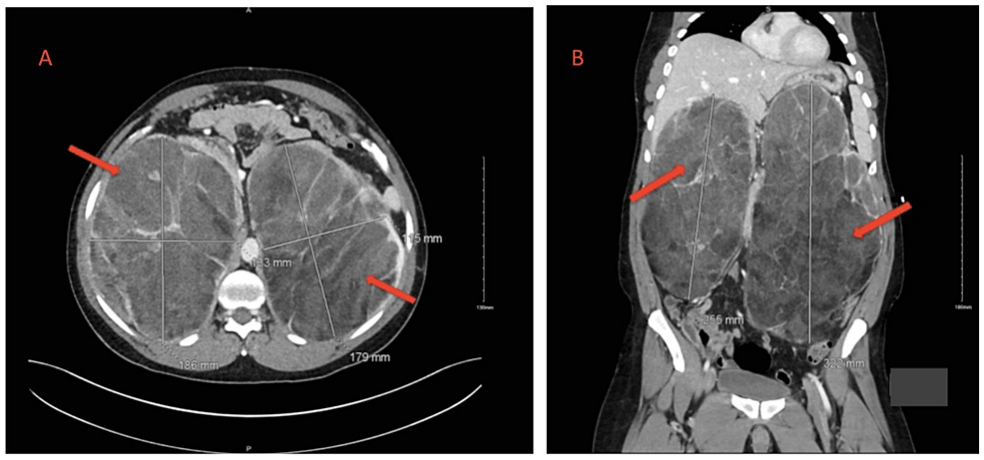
Axial (A) and coronal (B) views of the CT scan demonstrate bilateral adrenal masses (arrows) that are predominantly fatty components interspersed with areas of higher attenuation inside, consistent with giant myelolipomas. CT: Computed tomography.
